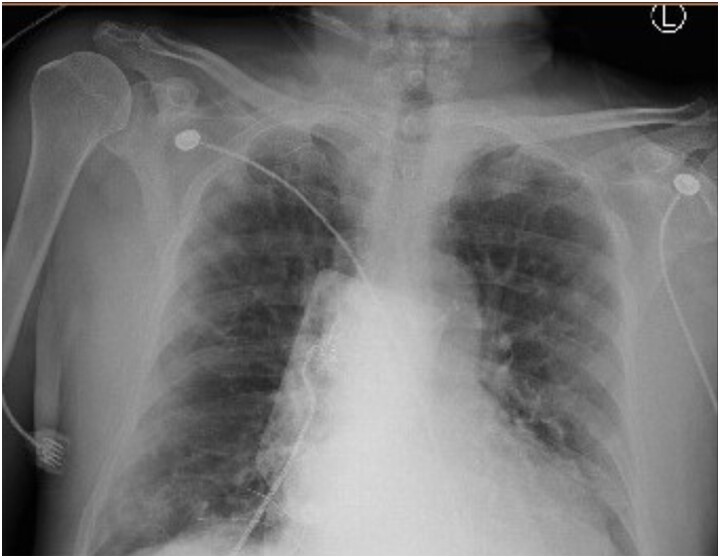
Chest x-ray showing bilateral peripheral patchy infiltrates consistent with known COVID-19 infection.
